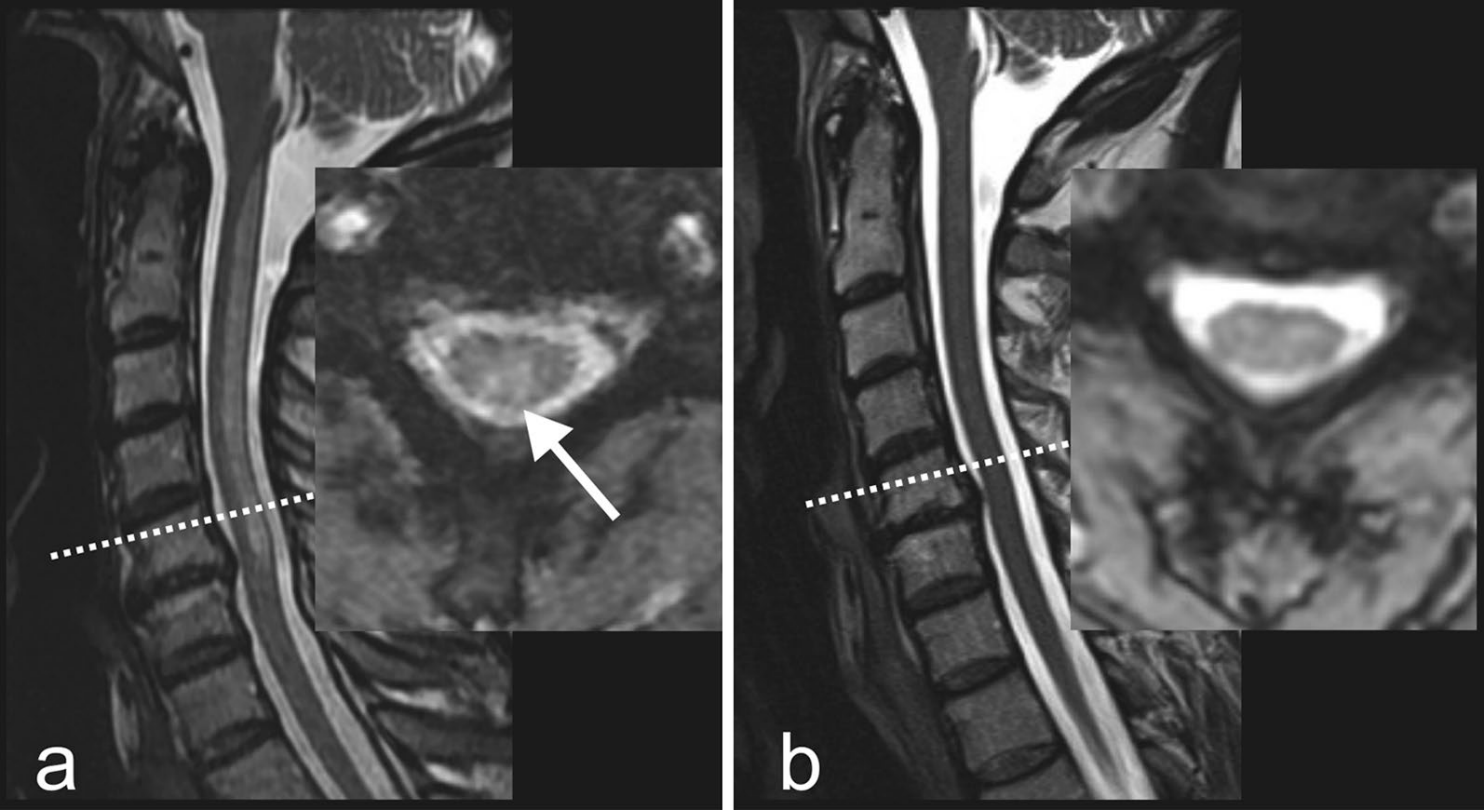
Magnetic resonance images of a patient suffering from subacute combined degeneration of spinal cord (SACD) before and after cobalamin substitution. a Before therapy: Sagittal and transversal T2-weighted images reveal an intraspinal hyper intensity of the dorsal cervical spinal cord (→) with no mass effect. No contrast enhancement of the lesion was found in T1-weighted images. b 5 months after the onset of cobalamin substitution: The hyper intensity completely disappeared. Known osteochondrosis and disc protrusion C5/6. In the sagittal view the transversal section plane is marked by a *dotted line*
